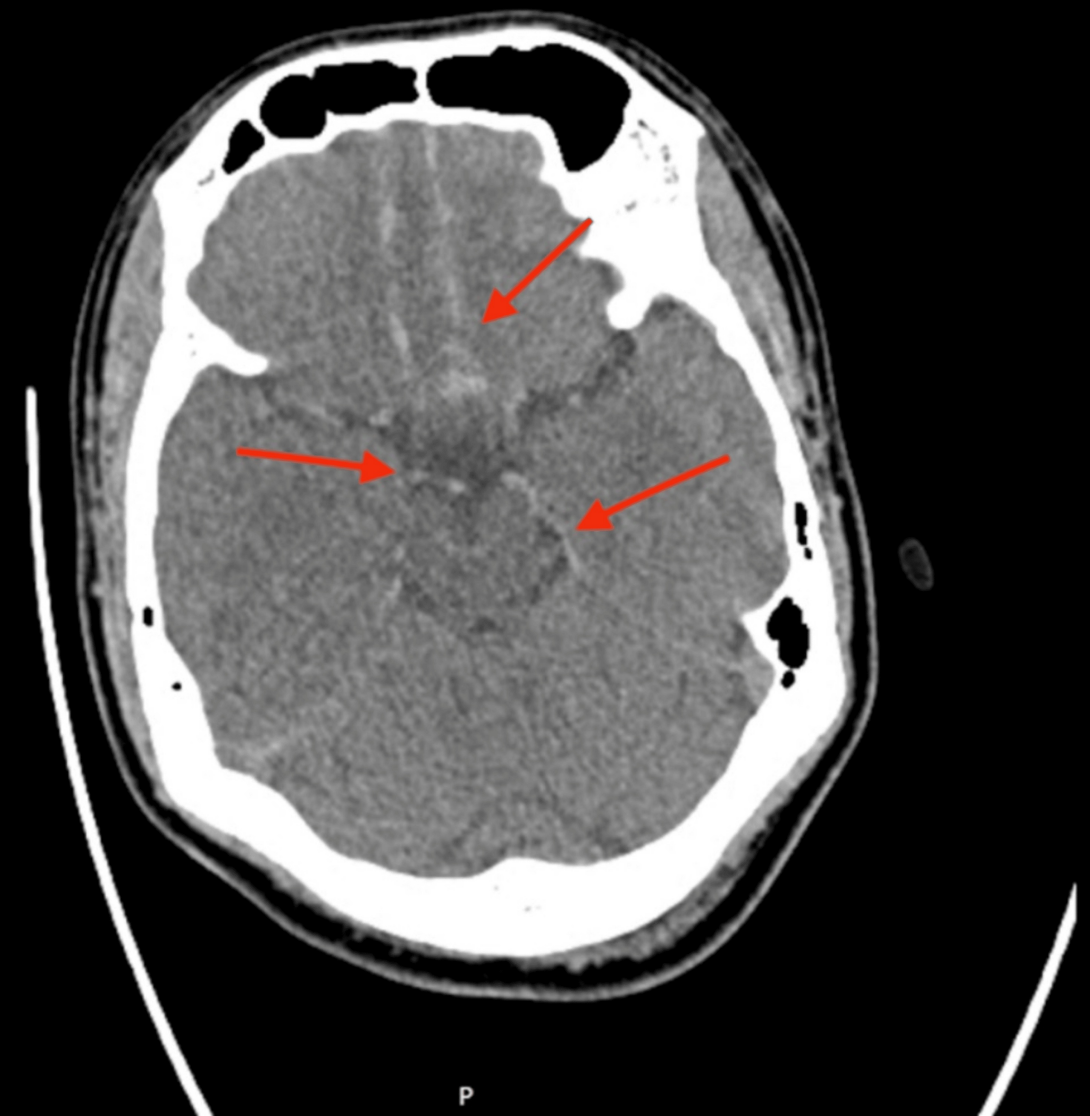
Subarachnoid hemorrhage Axial noncontrast CT brain demonstrating acute subarachnoid hemorrhage within the suprasellar cistern, extending over the inferior frontal cortex and along the falx cerebri (red arrows)
